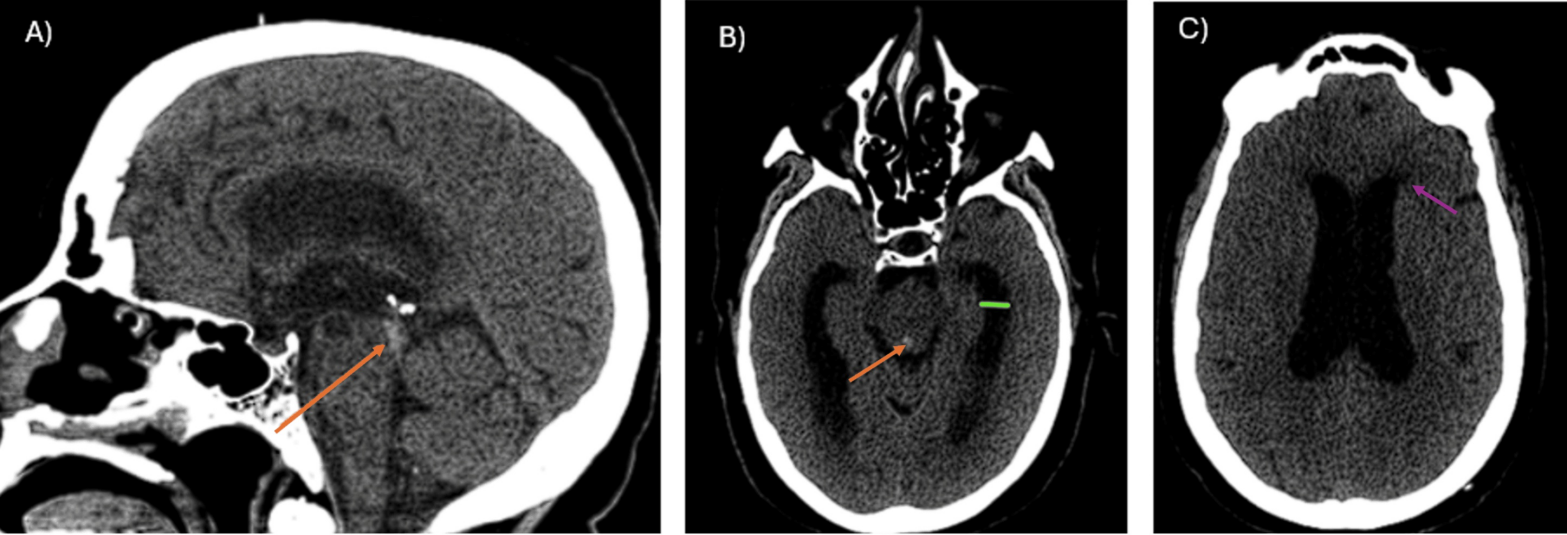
Non-contrast CT images. (A) Sagittal non-contrast CT image obtained at the onset of clinical symptoms, demonstrating a clot (orange arrow) with a density of 60 HU, located within the mesencephalic aqueduct. (B, C) Axial CT images obtained at the onset of clinical symptoms, showing enlargement of the bodies of both lateral ventricles and temporal horns (green line), as well as transependymal edema (purple arrow). A clot is identified within the mesencephalic aqueduct (orange arrow), representing direct and indirect signs of obstructive hydrocephalus.
